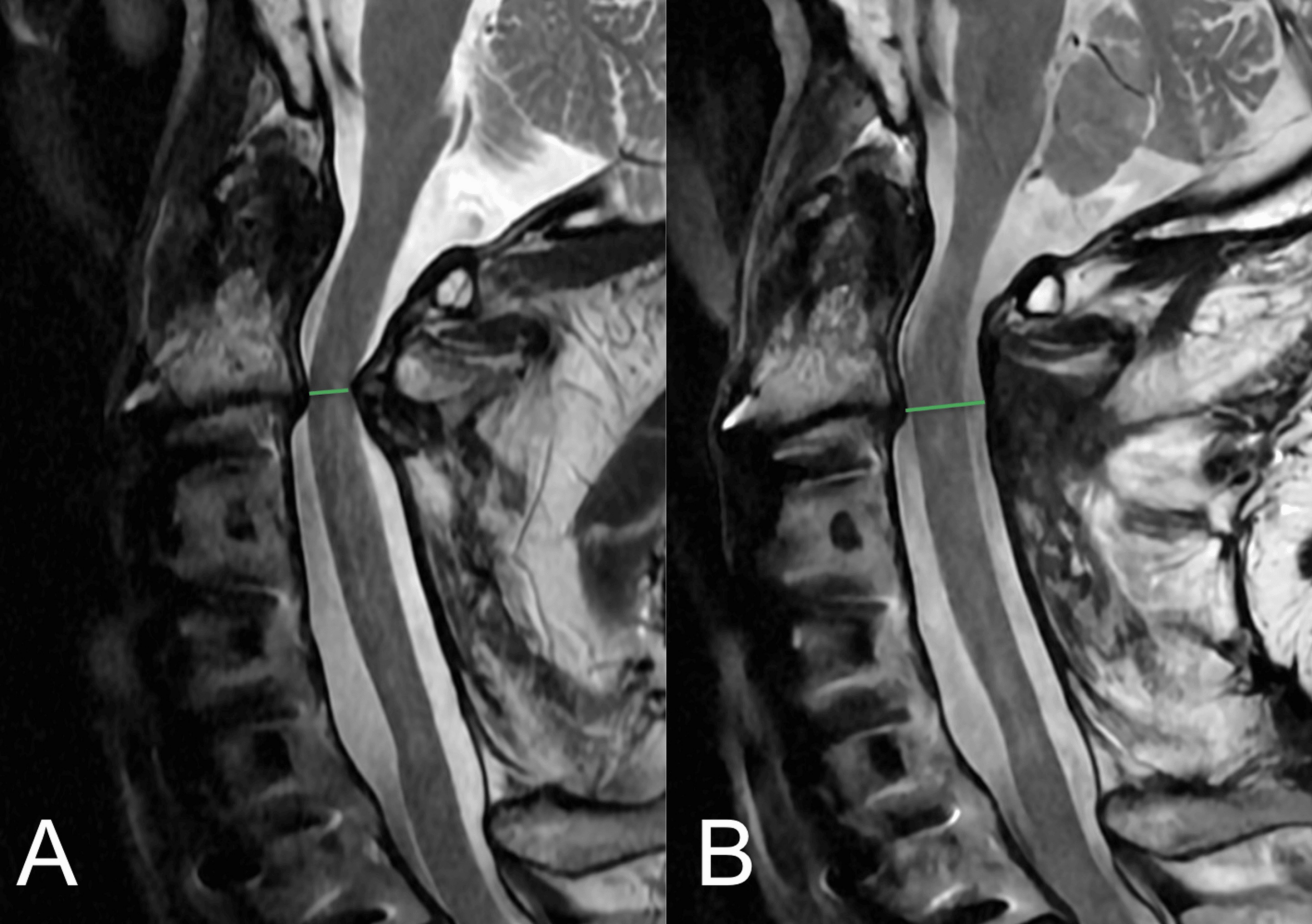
(A) Preoperative and (B) postoperative sagittal MRI images showing good decompression and removal of a primarily dorsally compressive ligamentum flavum. The green lines denote the AP central canal distance measured from the posterior disc in an orthogonal perpendicular line to the dorsal dura.
